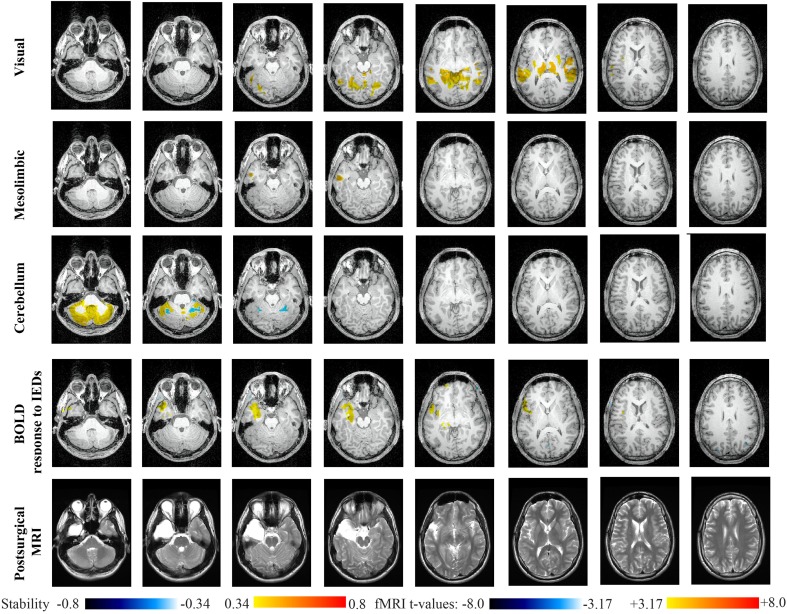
Evaluation of DANI on Patient 3 with left MTLE. Results are presenting most salient stability changes observed in *Cmap*_*n*_ followed by a t-map of the BOLD response to epileptic discharges and a postsurgical T2 MRI, all resampled in the native anatomical MRI space of the patient. Most salient stability changes observed for *Cmap*_*n*_ of Visual, Mesolimbic and Cerebellum networks are presented. The Visual and Cerebellum networks were also involved in significant interactions of modularity changes at *p* < 0.001. Same colormap conventions than in Figure 6.
